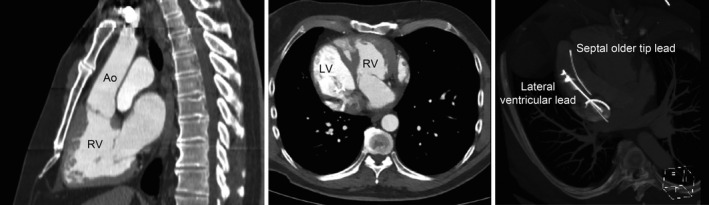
A chest CT angiogram revealed atrioventricular and ventriculoarterial discordance with the anatomic left ventricle (LV) in a “pulmonary” position and the anatomic right ventricle (RV) in a “systemic” position. The new ventricular lead was in a lateral position. Ao refers to the aorta.
